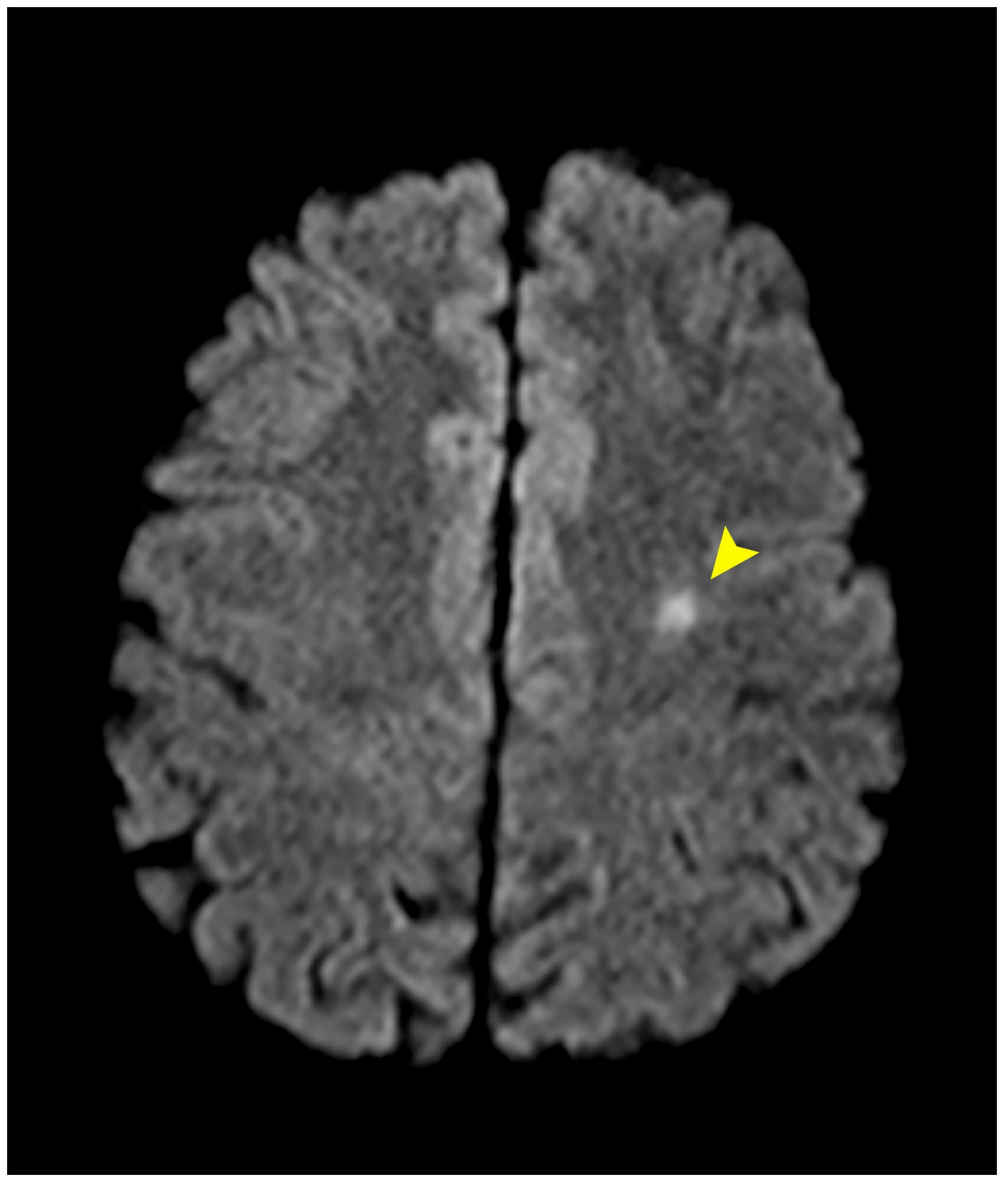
Axial MRI-DWI sequence demonstrates a small hyperintense lesion in the left centrum semiovale (arrow), exhibiting features consistent with acute ischemia.
